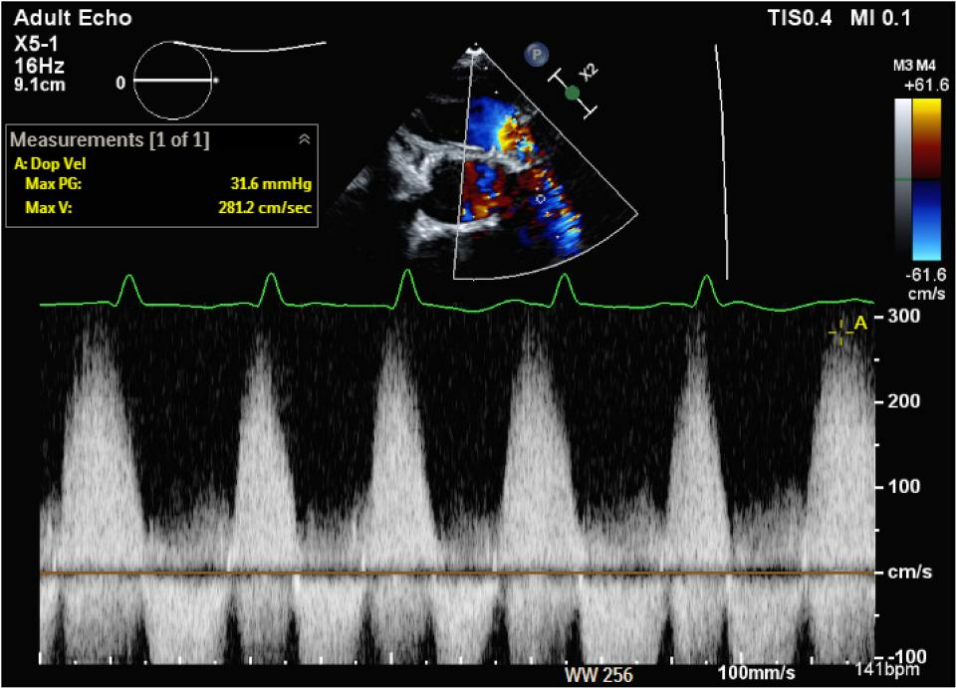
Continuous Doppler profile across the bioprosthetic pulmonic valve demonstrating a dense pulmonary regurgitant jet with a peak velocity of 2.8 m/s.
